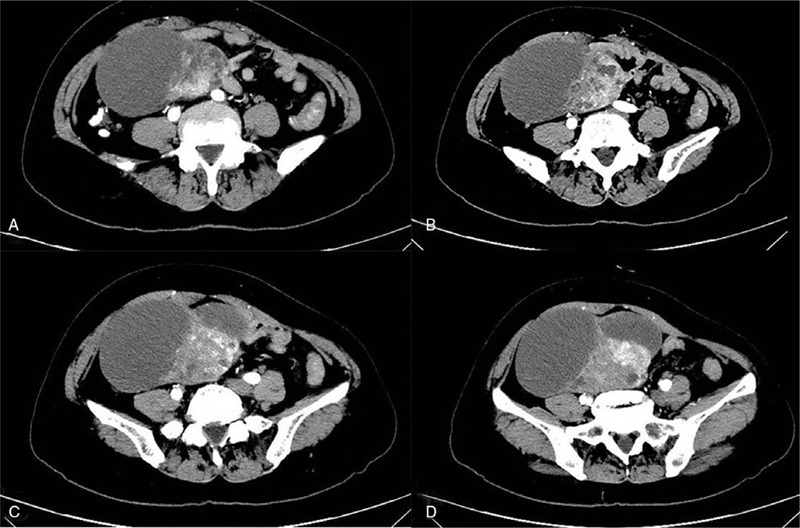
CT scan showed pelvic space-occupying lesions, which may be malignant ovarian tumors.
